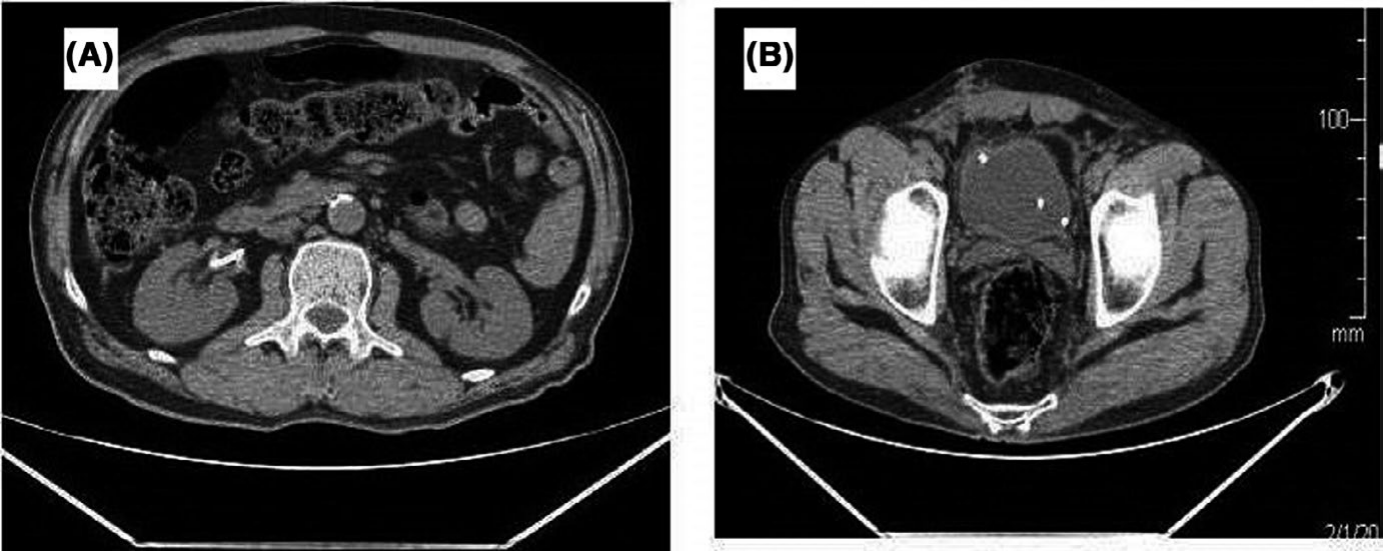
(A, B) Postoperative CT‐KUB revealed the double J in situ and high level of urinary bladder due to Psoas hitch
